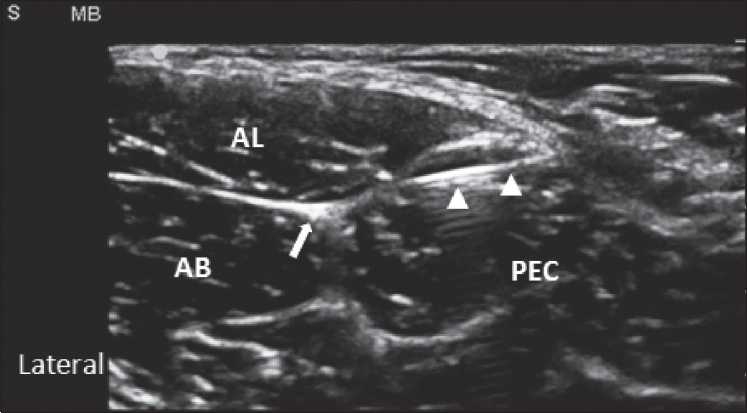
Ultrasound view of obturator nerve. PEC: pectineus muscle; AL: adductor longus muscle; AB: adductor brevis muscle; Arrow points at the anterior branch of the obturator nerve; triangles points at the needle
